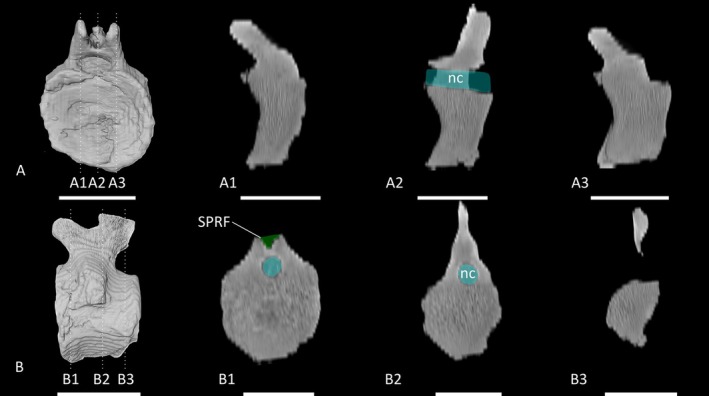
CT scan of *Pilmatueia faundezi* middle caudal vertebra MLL‐Pv‐016. (A, B) 3D rendering (A) anterior view, (B) left lateral view; (A1–A3) CT scan slices in parasagittal cross‐section, (B1–B3) CT scan slices in transverse cross‐section. nc, neural canal; SPRF, spinoprezygapophyseal fossa. Key: Blue highlight, neural canal; green highlight, neural spine fossa. Scale bars = 100 mm.
